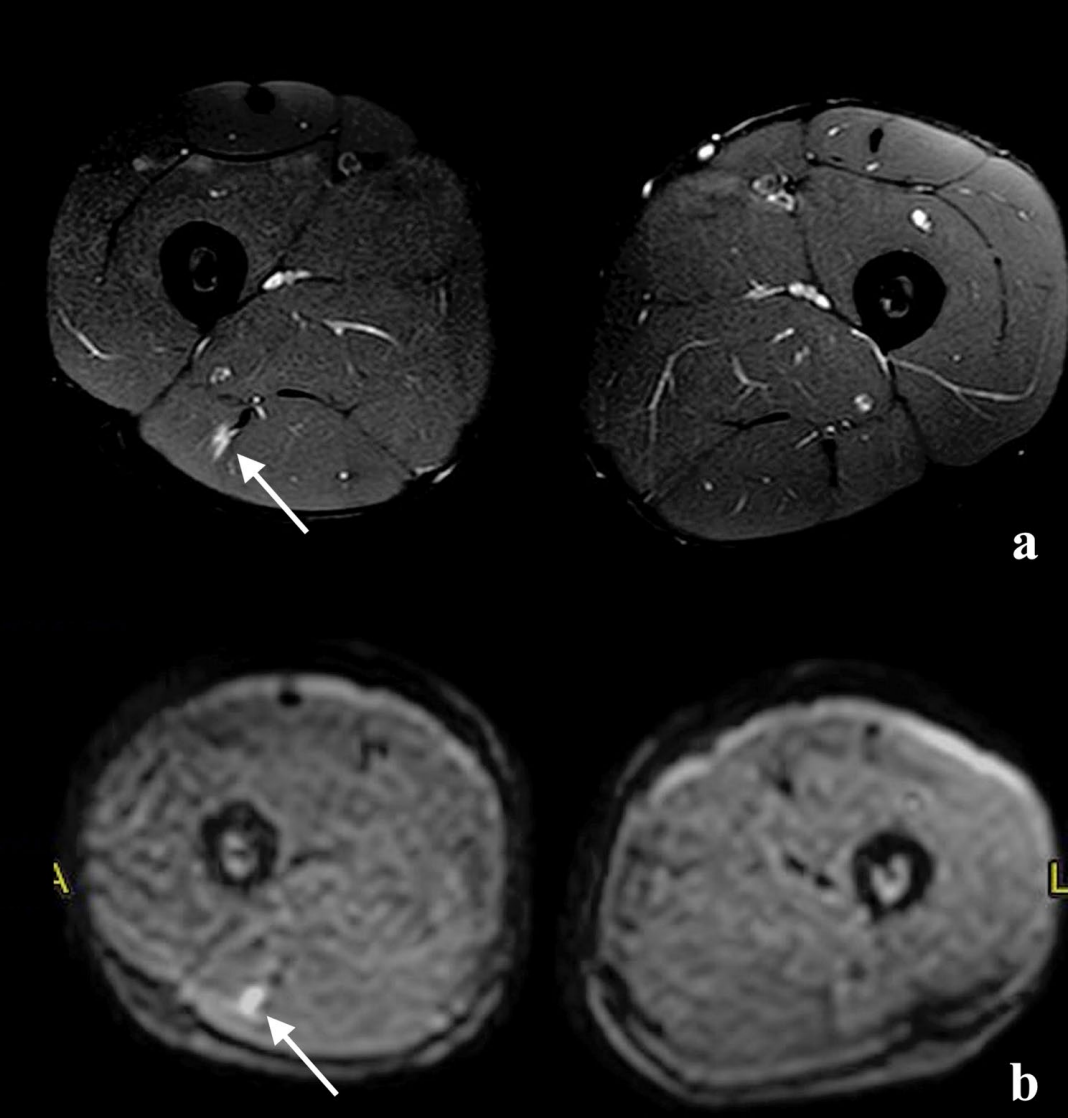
a Image obtained with DP TSE sequence with fat suppression shows 3A lesion (white arrow). b At the same anatomical region in the DWI study (b = 450), a clear signal hyperintensity is recognisable at the point where the greatest number of muscle fibres were interrupted (white arrow)
